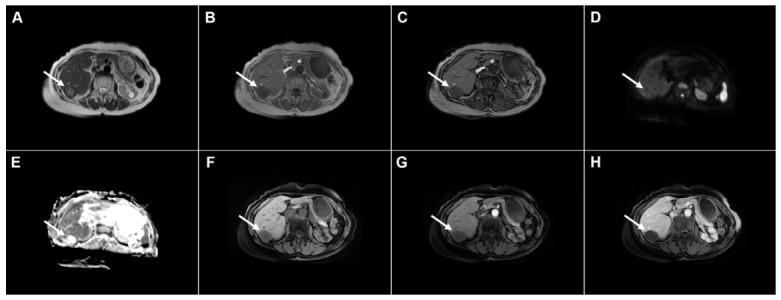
Cystic echinococcosis, WHO stage CE4, in a 68-year-old woman. Axial T2-weighted image (A) demonstrates a heterogeneous, semi-solid lesion (*arrow*) located posteriorly in liver segment VI. In-phase (B) and out-of-phase (C) images show that the lesion (*arrow*) is hypointense. No restricted diffusion can be observed (*arrow*) in the diffusion-weighted image (D) or in the corresponding ADC map (E). T1-weighted fat-suppressed images obtained before (F) and during the arterial (G) and portovenous phase (H) show no post-contrast enhancement, consistent with an avascular lesion (*arrow*).
